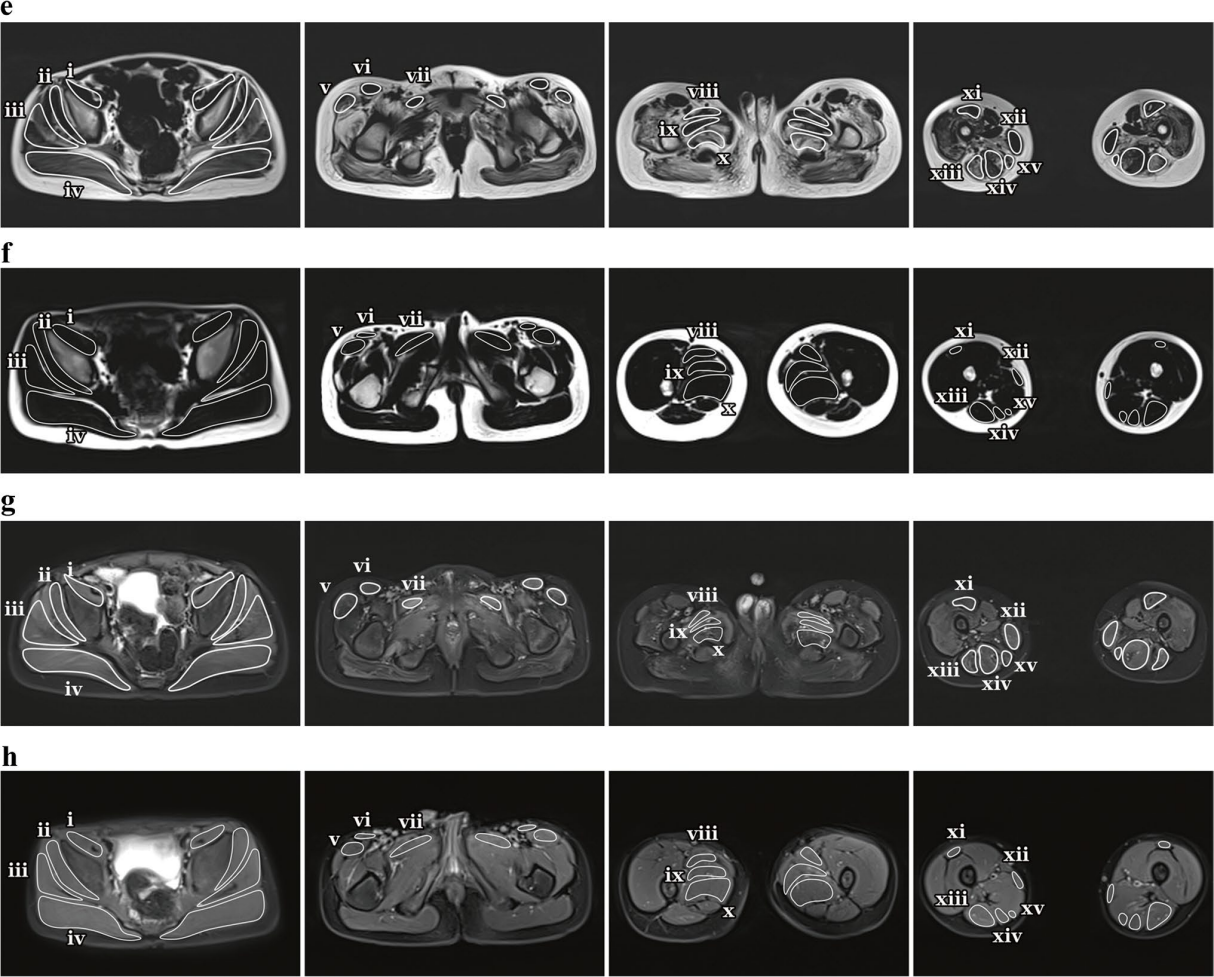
a–h Axial magnetic resonance imaging of the hip and pelvic muscles in a 10-year-old boy with Duchenne muscular dystrophy (a, c, e, g) and in an 8-year-old healthy boy (b, d, f, h). Sequences shown are T1 maps (a, b), T2 maps (c, d), Dixon (F) (e, f) and Dixon (W) (g, h). *i* iliacus, *ii* gluteus minimus, *iii* gluteus medius, *iv* gluteus maximus, *v* tensor fascia lata, *vi* sartorius, *vii* pectineus, *viii* adductor longus, *ix* adductor brevis, *x* adductor magnus, *xi* rectus femoris, *xii* gracilis, *xiii* biceps femoris, *xiv* semitendinosus, *xv* semimembranosus
